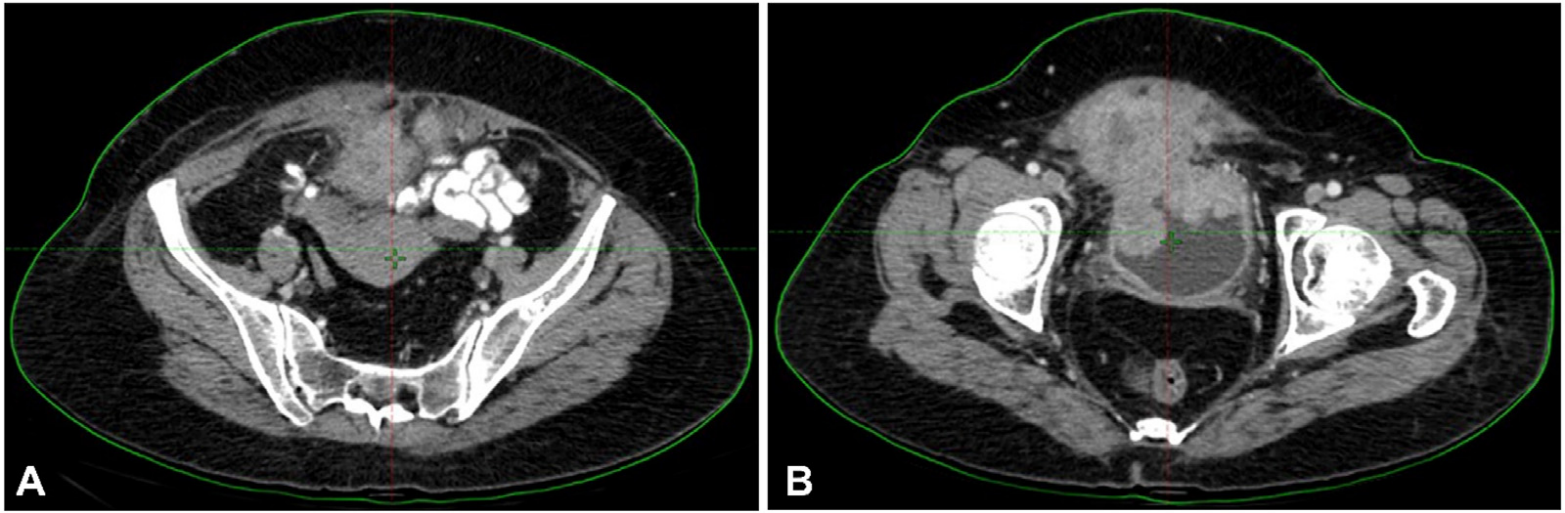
Diagnostic CT images showing pelvic mass involving the bladder.
